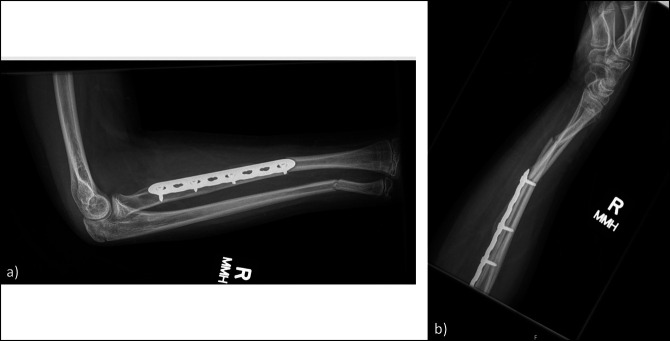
A, AP and (B) lateral radiograph of the right forearm of a 14-year-old boy demonstrating periprosthetic both-bone forearm fracture adjacent to the retained plate on the radius. The plate was placed 1 year earlier.
